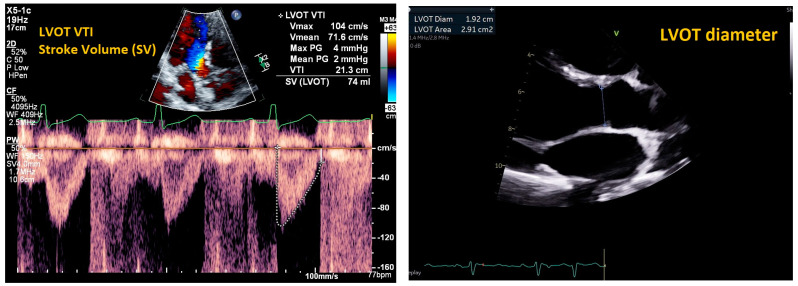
Measuring cardiac output (CO). CO = heart rate [HR] × stroke volume [SV]. Left panel: left ventricular outflow tract velocity time integral (LVOT VTI) in apical four chamber view. SV = *π* × R^2^ × (LVOT VTI). Right panel: diameter of the LVOT measured in parasternal long axis view in systole.
